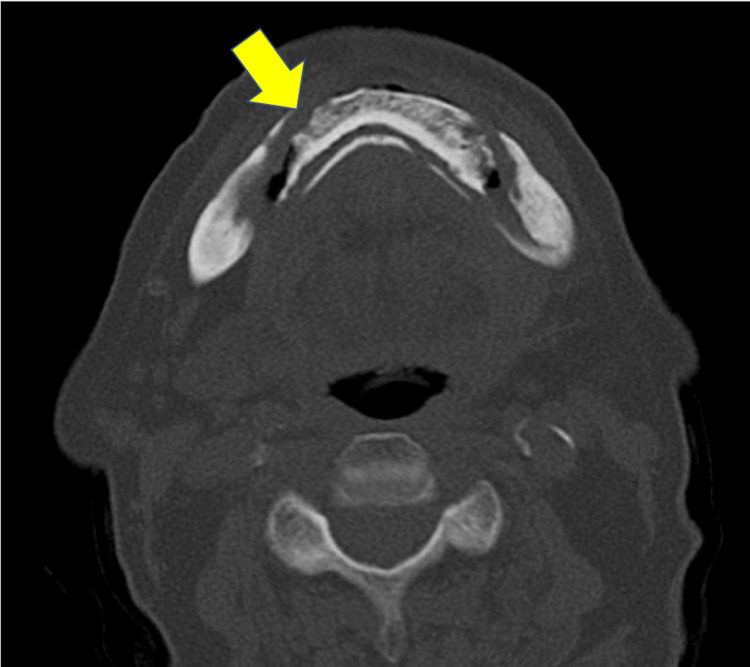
Radiographic finding nine months after the initial visit. Axial MDCT image showed a trend toward further separation of the sequestrum compared with the second one (the yellow arrow indicates the signs of separation of the sequestrum). MDCT, multidetector computed tomography
